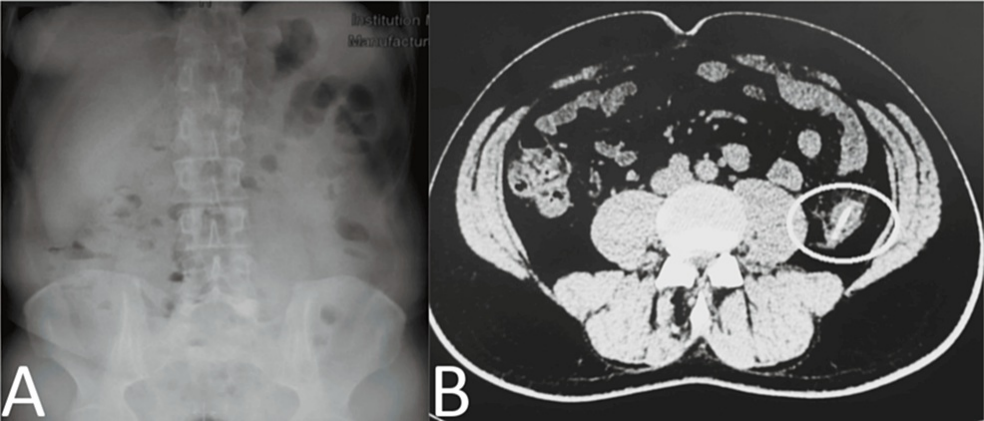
Abdominal X-ray and CT of the patient. (A) Abdominal X-ray revealed no abnormal findings, including the absence of free intra-peritoneal air. (B) CT imaging findings of foreign body penetration into the descending colon.
